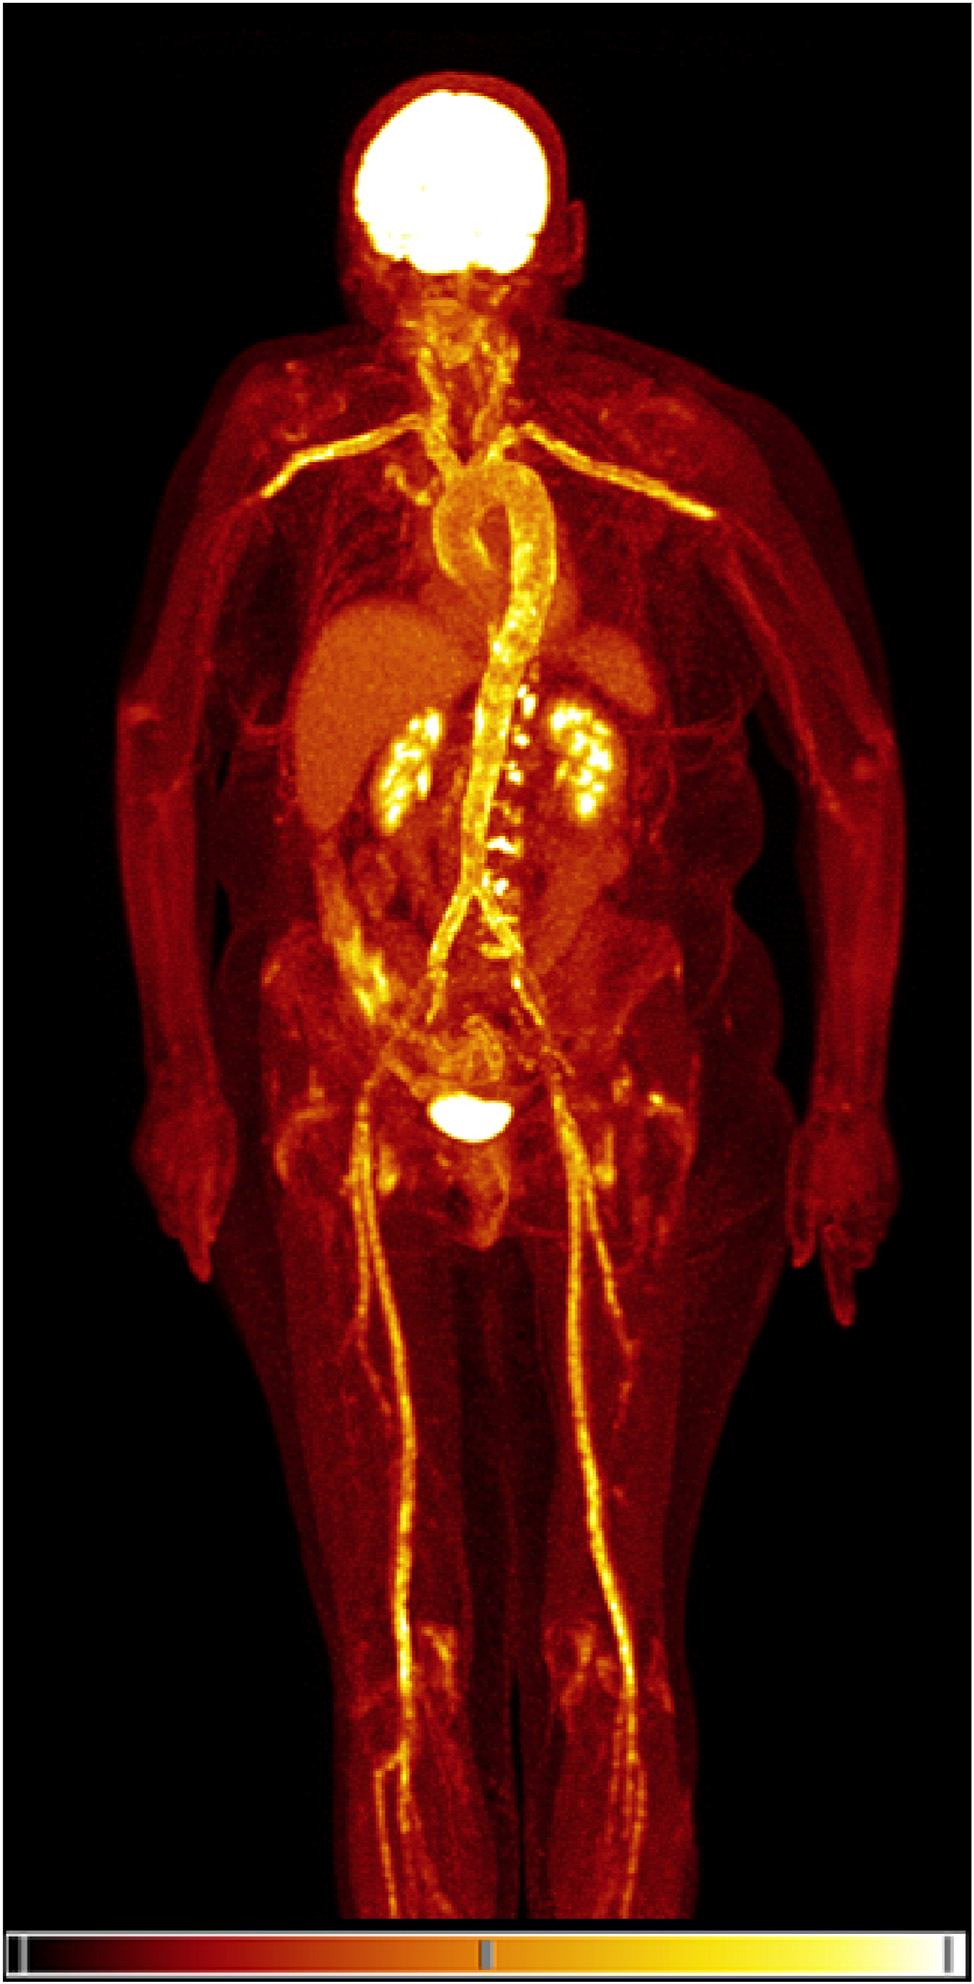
MIP View FDG-PET (Biograph Vision Quadra) scan in a 69-year-old female with lvGCA and PMR of the shoulders, hips, knees, and lumbar spine. MIP, maximum intensity projection; FDG-PET, [18F]-fluoro-2-deoxy-d-glucose positron emission tomography/computed tomography; lvGCA, large vessel GCA; PMR, polymyalgia rheumatica.
